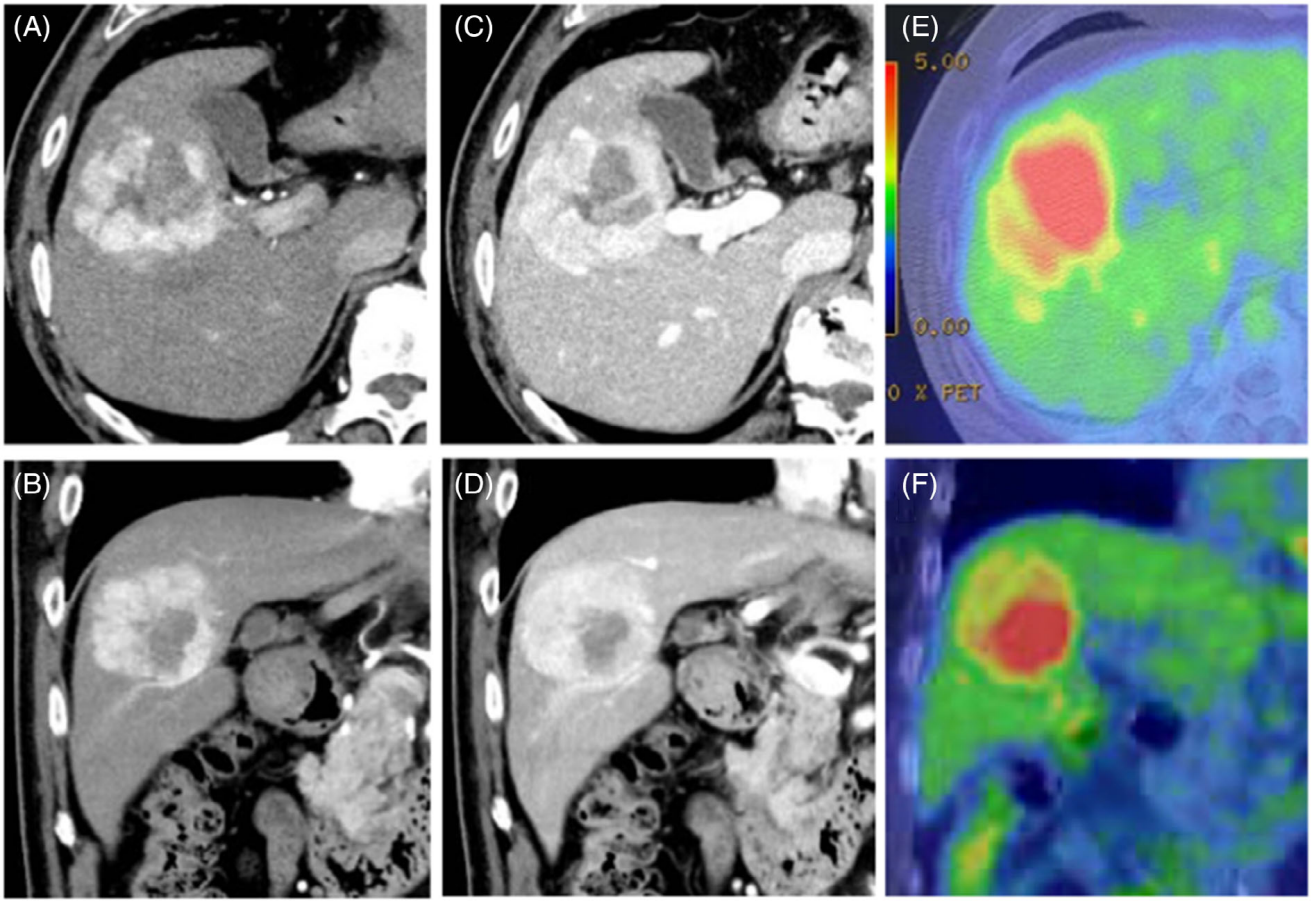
Findings of enhanced abdominal computed tomography (CT) and F‐18 fluorodeoxyglucose (FDG)‐positron emission tomography (PET)/CT: Abdominal CT showing a hepatic mass with early enhancement of the peripheral areas in the arterial phase (A, B) and low‐density structures within the tumor in the delayed phase (C, D). FDG‐PET/CT revealing heterogeneous uptake in the tumor (E, F).
